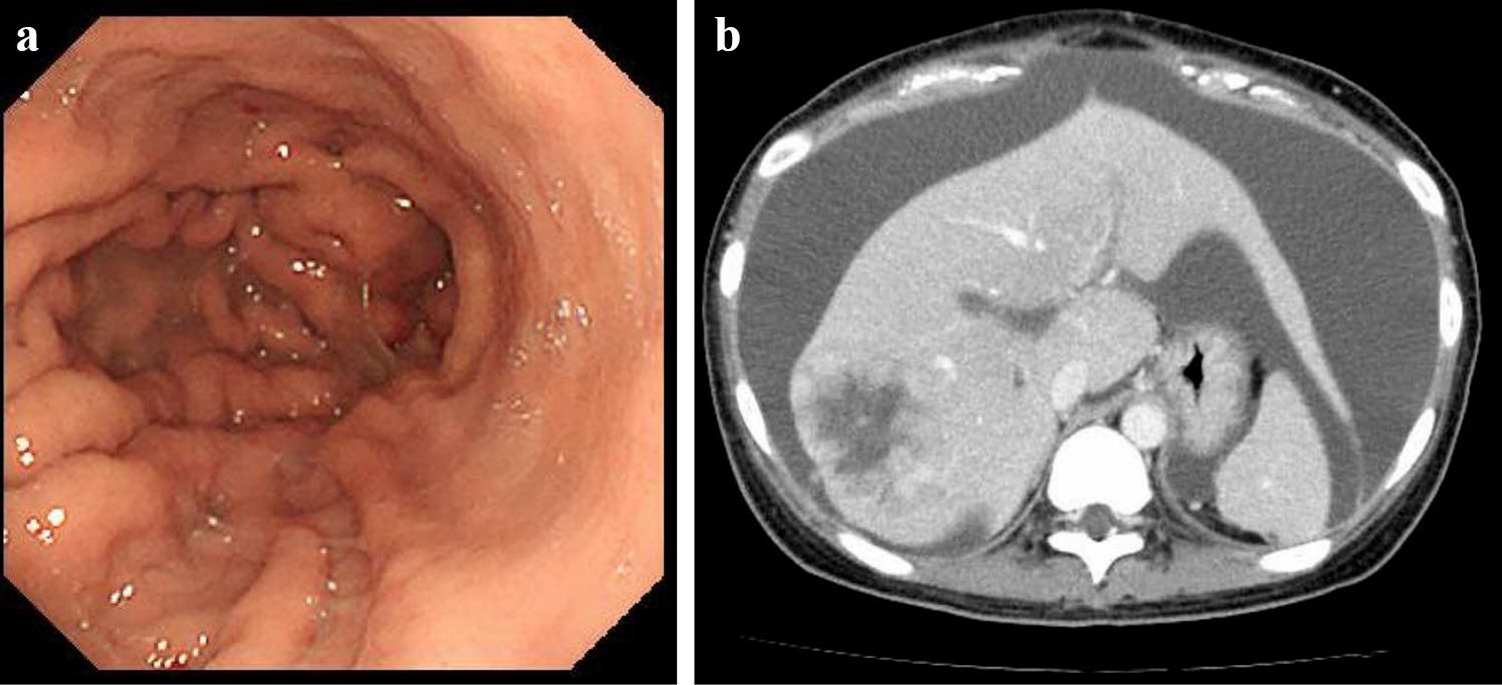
Gastroscopy and abdominal CT after chemotherapy. a After the chemotherapy, mucosal swelling, and thick folds were diminished and ulceration was reduced in size on gastroscopy. b The hepatic tumor decreased in size on the CT
